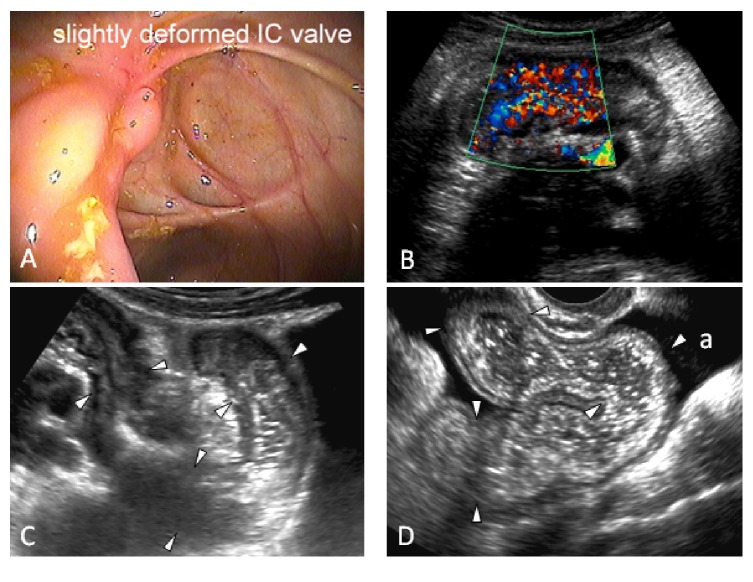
Stricture. 27-year-old female with no prior diagnosis of Crohn's disease with a failure to intubate the terminal ileum on colonoscopy due to a deformity at the ileocecal (IC) valve shown in (A). (B) An ultrasound image of the terminal ileum shows that it is thick-walled and hyperemic. (C) Additional ultrasound image shows a fixed acute angulation of the bowel. (D) shows the bowel proximal to the angulation with increased calibre and increased fluid content. There is ascites (a). Interpretation: hyper- and dysfunctional peristalsis on real-time imaging indicated acute inflammatory stricture with incomplete mechanical bowel obstruction. Biological therapy was initiated after ultrasound.
